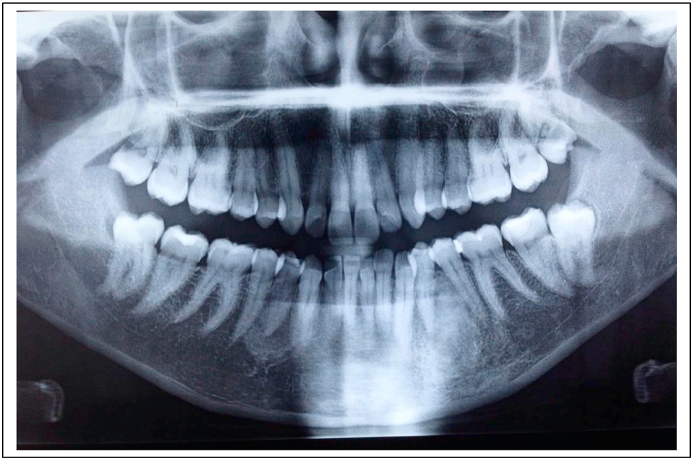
Preoperative radiograph showing the impacted mandibular left third molar.
